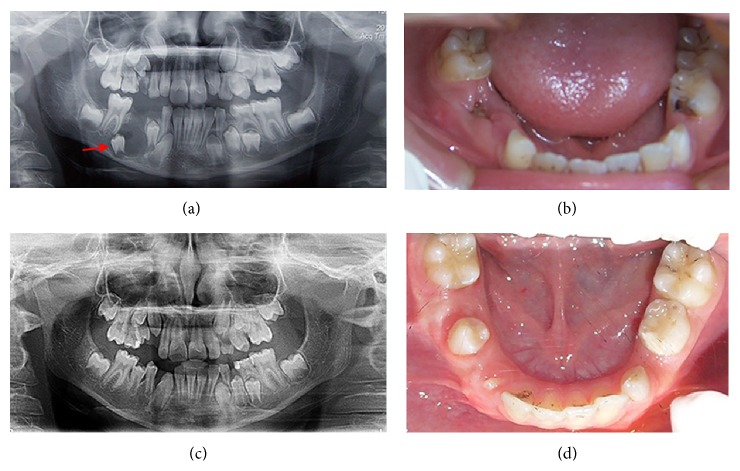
Case 1: an 8-year-old boy. (a) Initial panoramic X-ray image showing a DC in the right mandibular region. (b) Intraoral view of the cystic region. Tooth 85 had been extracted 1 week before the initial examination. (c)-(d) 16-month follow-up X-ray image and intraoral photos showing the complete healing of the cystic region and eruption of the affected tooth.
